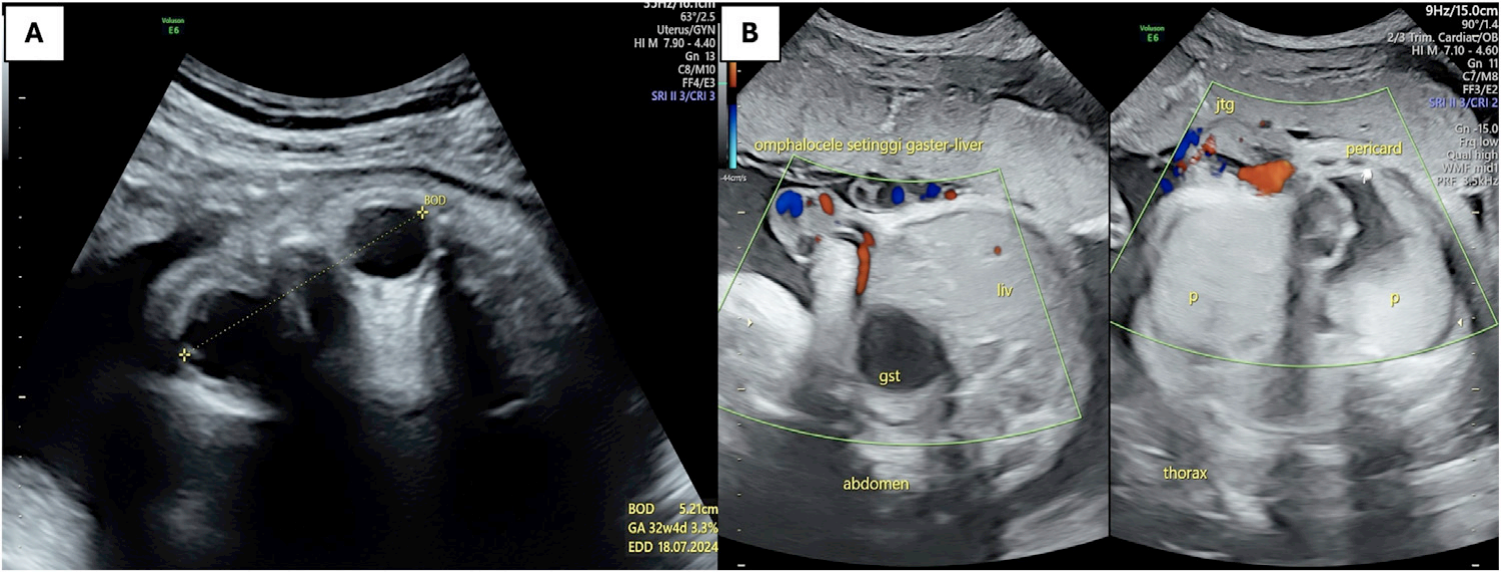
(A) Ultrasound show binocular distance 5.21 cm result to hypotelorism and (B) supraumbilical omphalocele at the level of the stomach-liver.
